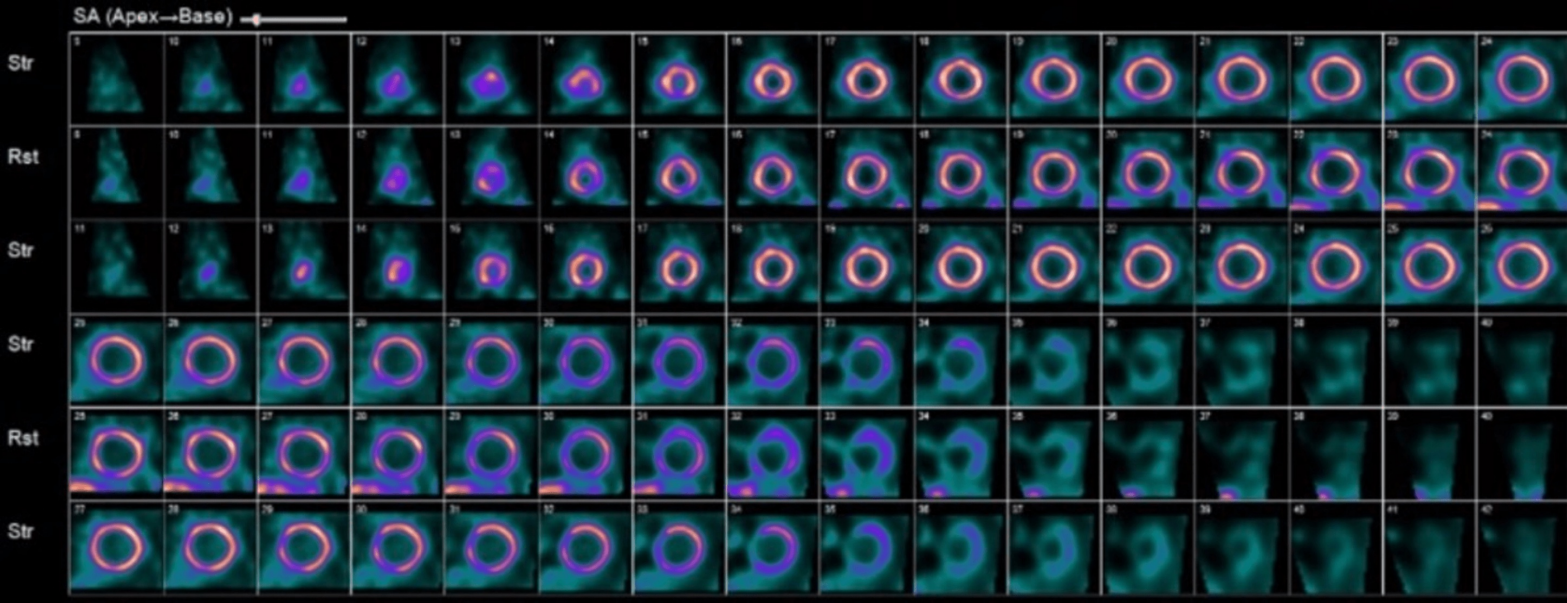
Nuclear stress test with gated tomography (parasternal short-axis view) showing homogenous tracer uptake, global left ventricular dysfunction, diffuse hypokinesis, and an LVEF of 25%. LVEF: left ventricular ejection fraction
